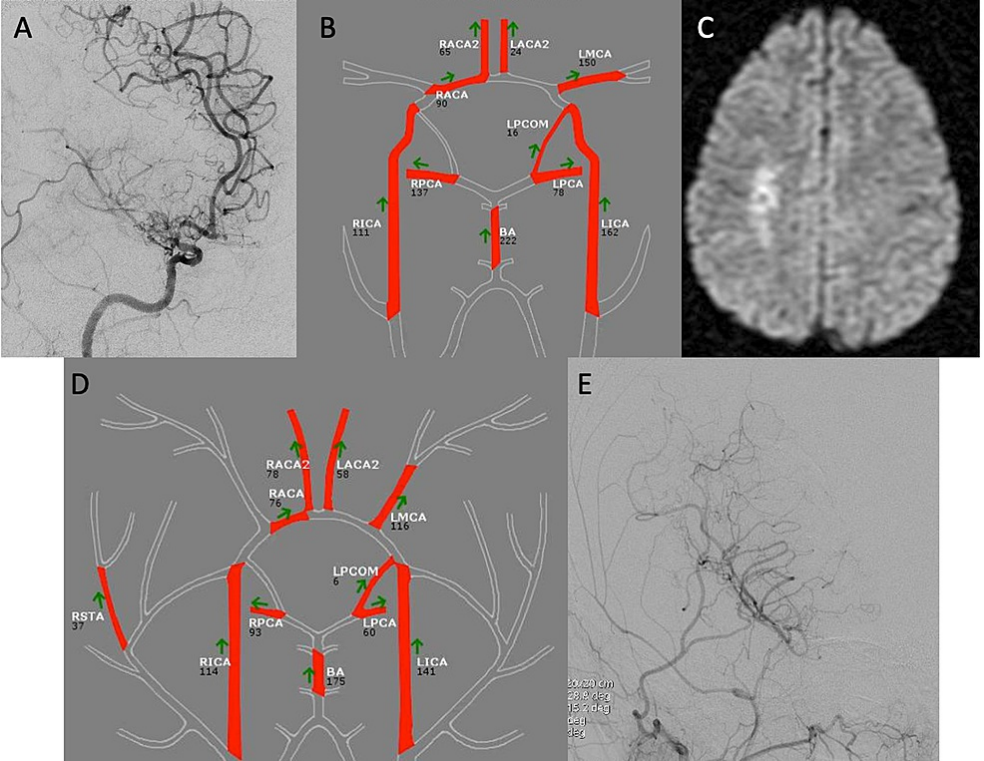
Case example Imaging of an example patient undergoing surgical revascularization. (A) Preoperative angiogram demonstrating supraclinoidal ICA and MCA stenosis as well as early moyamoya collaterals with (B) MRA NOVA demonstrating no flow in the RMCA and (C) showing DWI restriction on initial MRI in a watershed region. The patient underwent right-sided STA to MCA bypass with (D) postoperative MRA NOVA showing bypass flow of 37 ml/min and (E) postoperative angiography of the external carotid artery demonstrating good flow in the bypass graft. ICA: internal carotid artery; MCA: middle cerebral artery; RMCA: right middle cerebral artery; DWI: diffusion-weighted imaging: STA: superficial temporal artery; MRA: magnetic resonance angiography; NOVA: Non-invasive Optimal Vessel Analysis; RACA2: right anterior cerebral artery A2; LACA2: left anterior cerebral artery A2; LMCA: left middle cerebral artery; RACA: right anterior cerebral artery; LPCOM: left posterior communicating artery; RPCA: right posterior cerebral artery; BA: basilar artery; LPCA: left posterior cerebral artery; RICA: right internal carotid artery; LICA: left internal carotid artery; RSTA: right superficial temporal artery.
